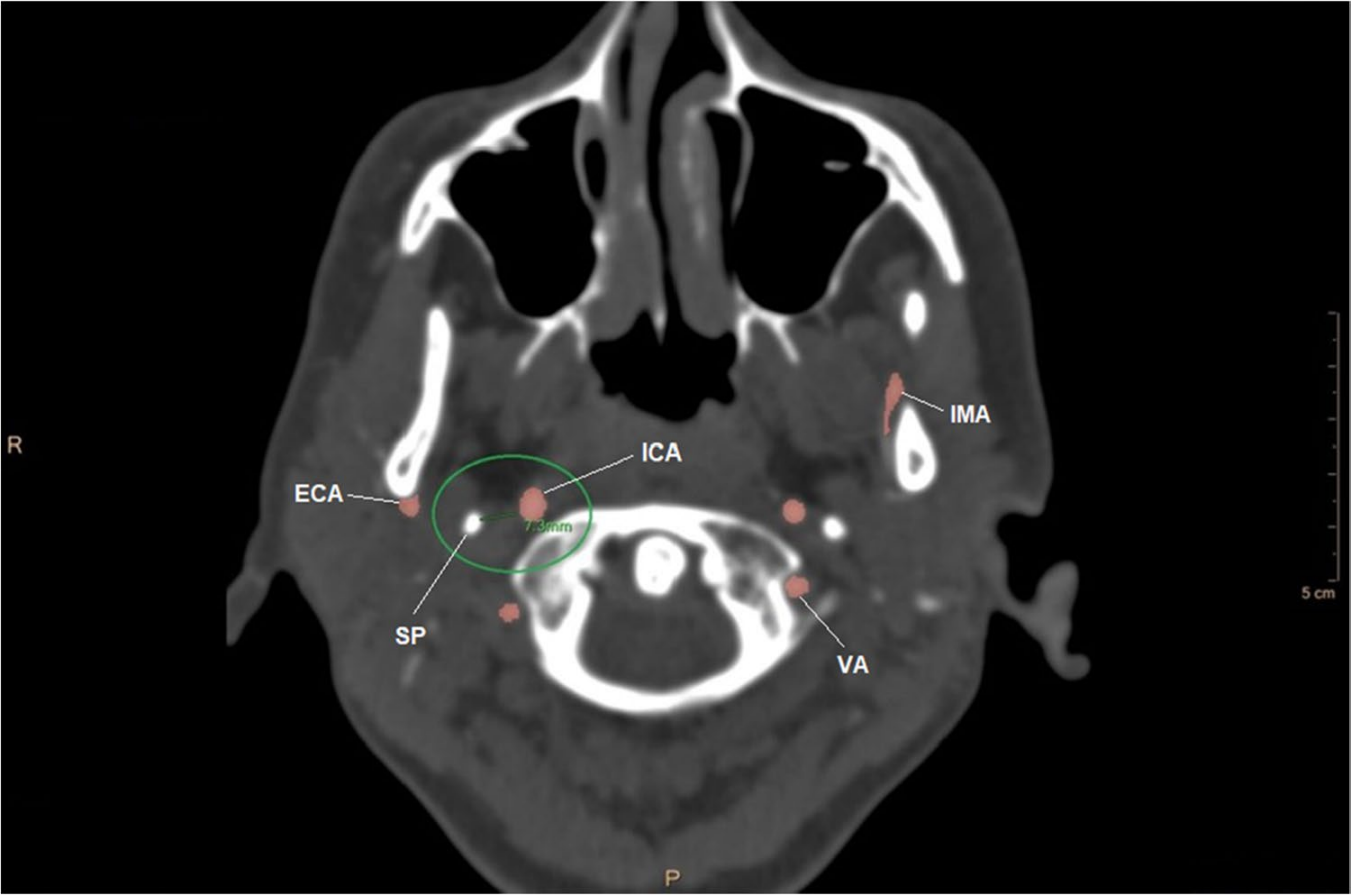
CT angiography image of marginal distance (SP, styloid process; ICA, internal carotid artery; VA, vertebral artery; IMA, internal maxillary artery)
